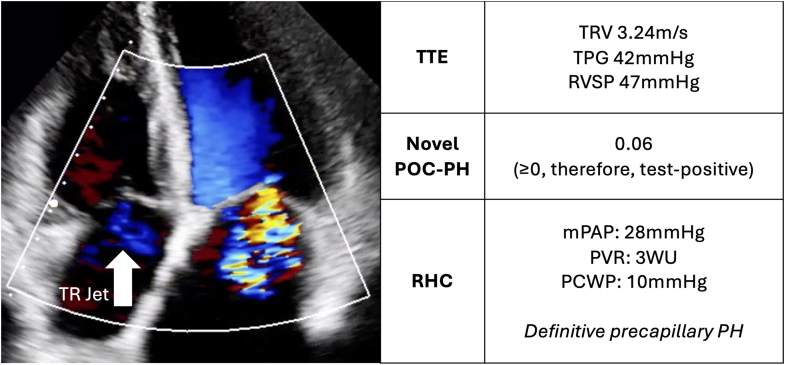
For a Single Case, TTE (With TR Jet Image and Measurements), the Novel POC-PH Test, and RHC, Resulting in Precapillary PH Diagnosis mPAP = mean pulmonary artery pressure; PCWP = pulmonary capillary wedge pressure; PH = pulmonary hypertension; POC-PH = point-of-care test for pulmonary hypertension; PVR = pulmonary vascular resistance; RHC = right heart catheterization; TPG = tricuspid pressure gradient; TR = tricuspid regurgitation; TRV = tricuspid regurgitant velocity; TTE = transthoracic echocardiography.
